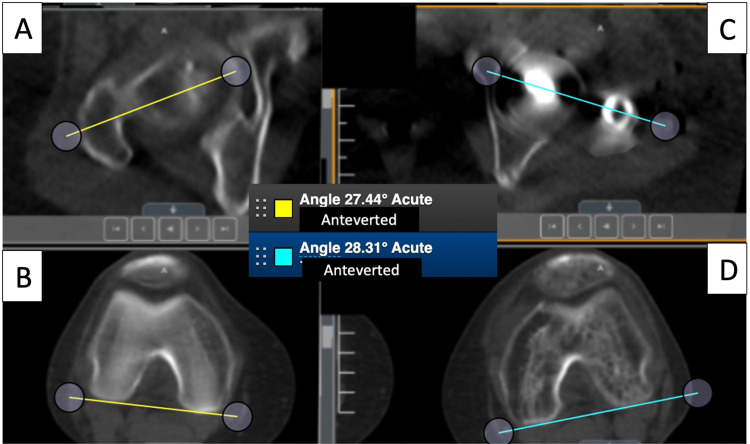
Post-correction CT scanogram of both femurs. Right femoral anteversion (A, B) is unchanged 27 degrees (yellow). The left femur (C, D) is measured to be in 28 degrees of femoral anteversion (light blue lines). There is a 0.75 deg difference between the two femurs which is in the acceptable range of <15 deg difference between the two sides. ° = degree
